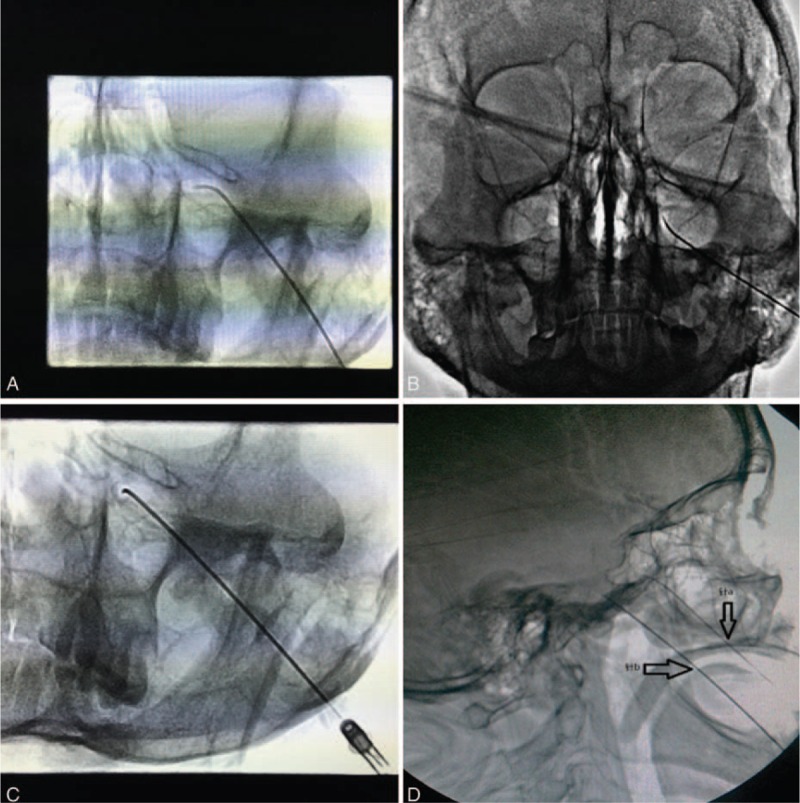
(A) Anteroposterior x-ray image showing the projection of the bilateral petrous pyramids onto the middle portion of the maxillary sinus. Foramen rotundum is found within the maxillary sinus superior to the petrous pyramid. (B) An anteroposterior view with the curved needle advanced through the posterolateral wall of the maxillary sinus toward foramen rotundum. (C) The needle was rotated in order for the tip to face posteriorly toward the foramen and advanced until through the foramen. (D) A lateral x-ray showing the difference between needle insertion at foramen ovale and foramen rotundum. The lower needle was advanced through foramen ovale using the Hartel method.
